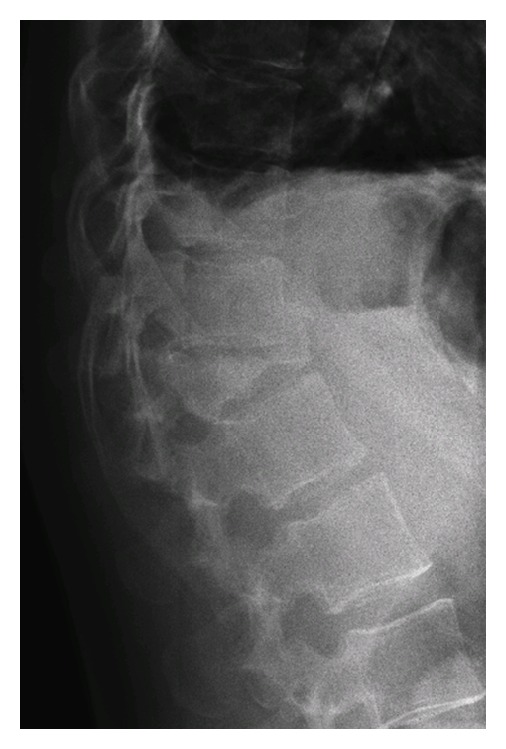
X-ray showing a burst fracture of T12.
